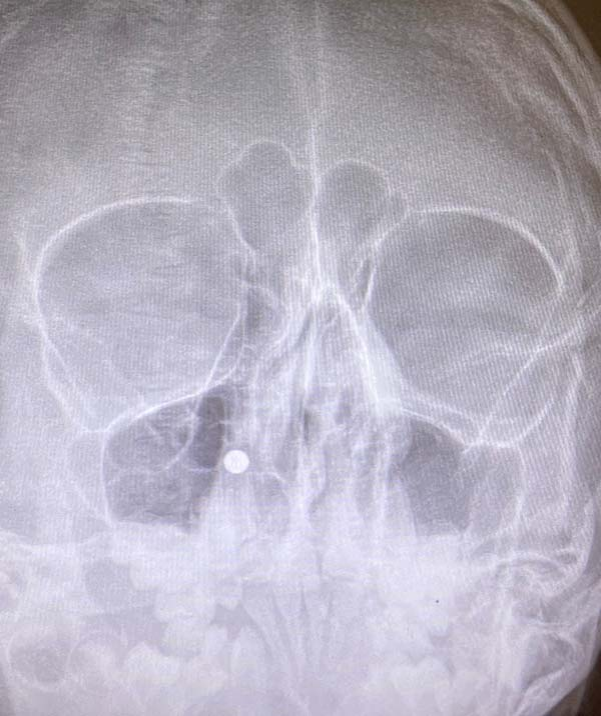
Coronal view X-ray of sinuses.
